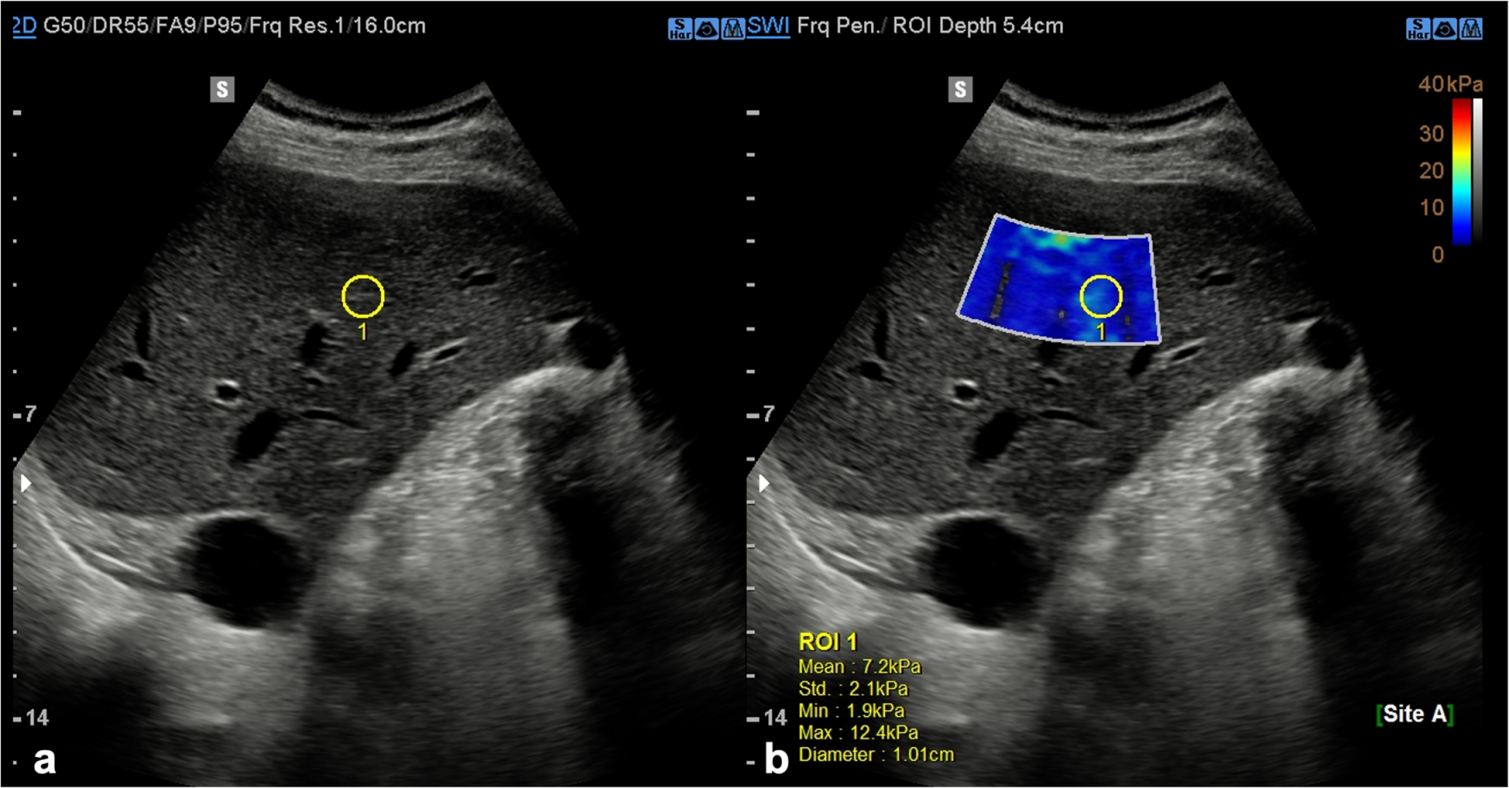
Ultrasound shear wave elastography for assessment of hepatic fibrosis. a Brightness-mode image with a 1.0-cm circular region of interest indicating a mean stiffness of 7.2 kPa. b Shear wave imaging mode indicating the liver stiffness with a color parametric map along a scale from 0 to 42 kPa
